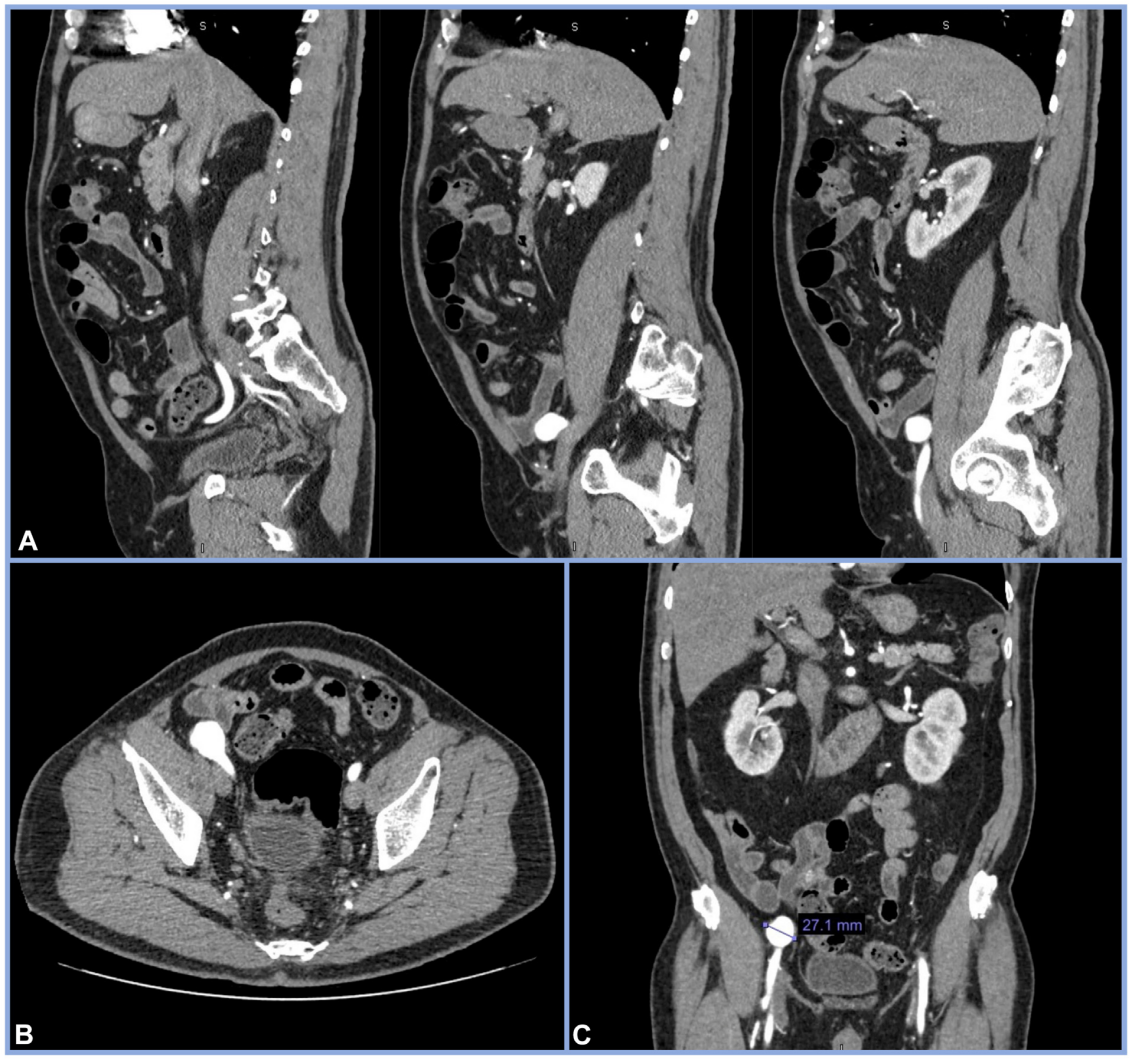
Preoperative computed tomography angiography showing an isolated aneurysm of the suprainguinal right external iliac artery (*EIA*). (A) Sagittal. (B) Axial. (C) Coronal with measurement.
